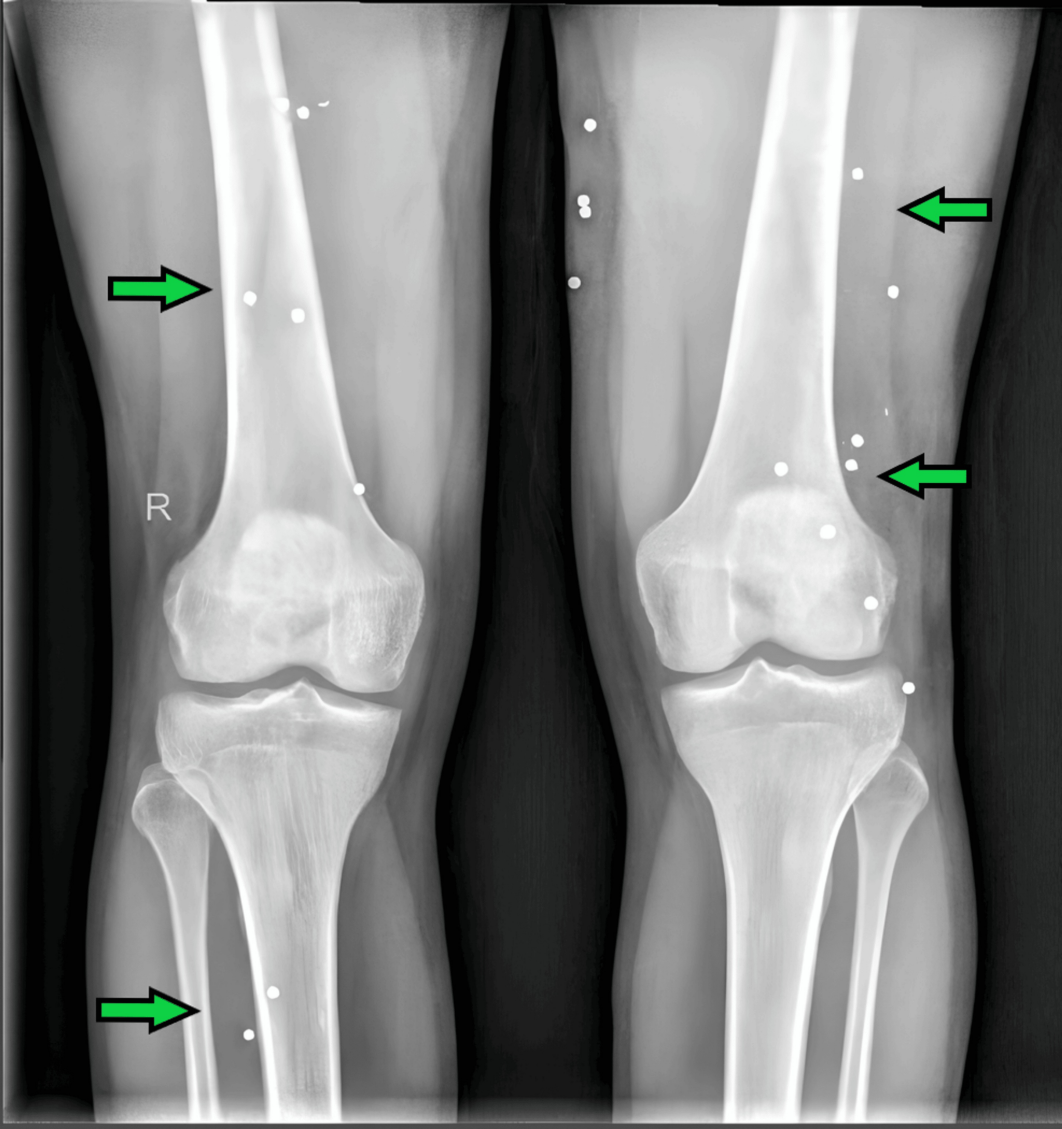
X-ray AP view of the bilateral knees with the distal thigh and upper leg showing multiple well-defined radiodense foreign bodies, which are reminiscent of tiny pellets AP: anteroposterior
